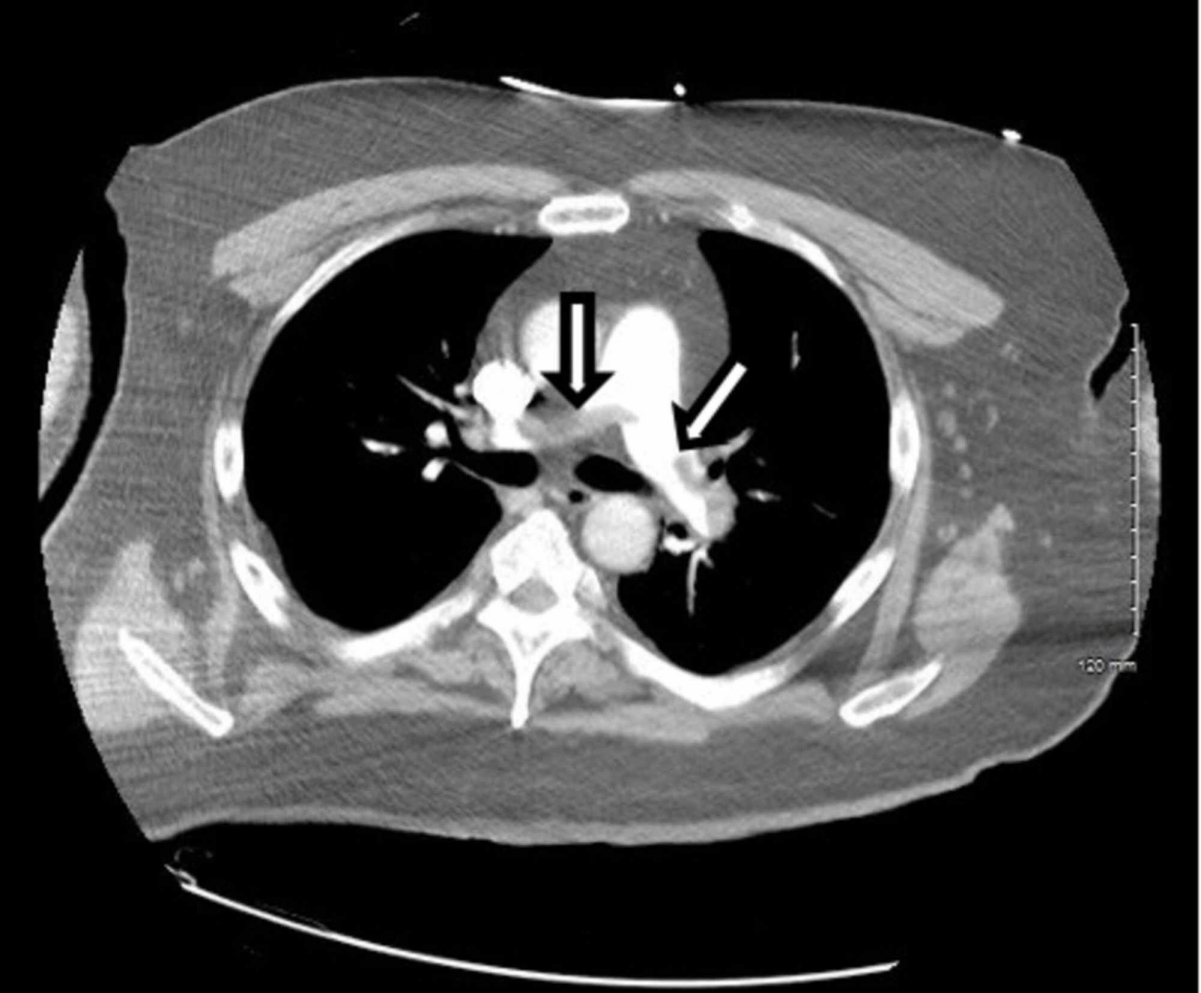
CTPA demonstrating a saddle embolus in the main pulmonary artery and emboli involving the right and left main pulmonary arteries (arrows). CTPA, computed tomography pulmonary angiogram.
